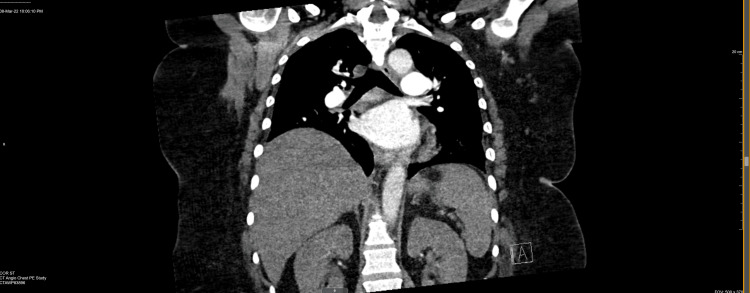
Computed tomography scan chest angiogram (coronal section) demonstrating right side diaphragm elevation
